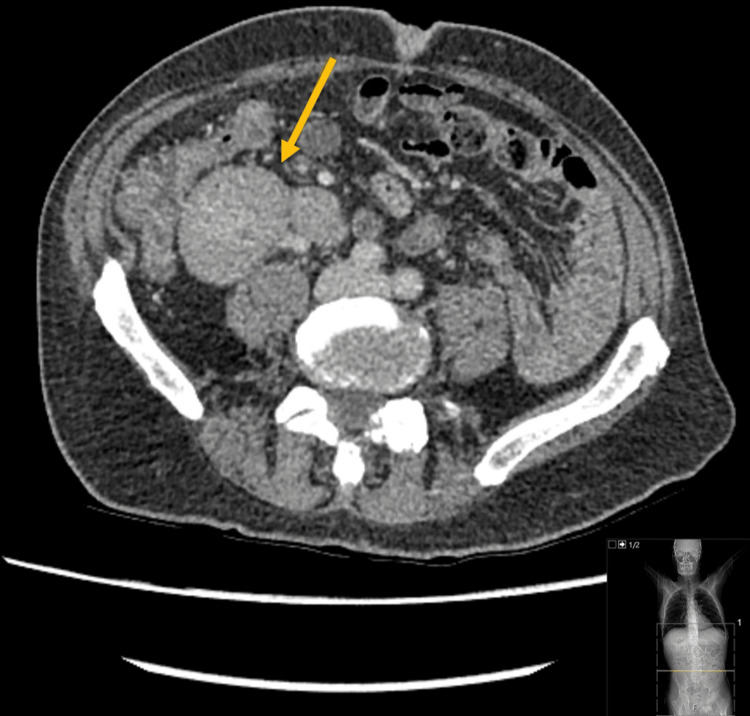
Cross-sectional CT image of the abdomen and pelvis obtained in April 2025 Cross-sectional CT image of the abdomen and pelvis demonstrating abnormally large mesenteric lymph nodes (yellow arrow).
